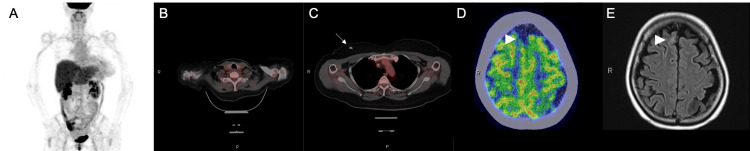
Imaging findings after six cycles of T-DXd PET-CT scan (A, B, C, D) and MRI of the brain (E). The white arrow points to the central venous port. The arrowhead points to the largest of the multiple brain metastases. T-DXd: Trastuzumab deruxtecan; PET-CT: Positron emission tomography/computed tomography; MRI: Magnetic resonance imaging
